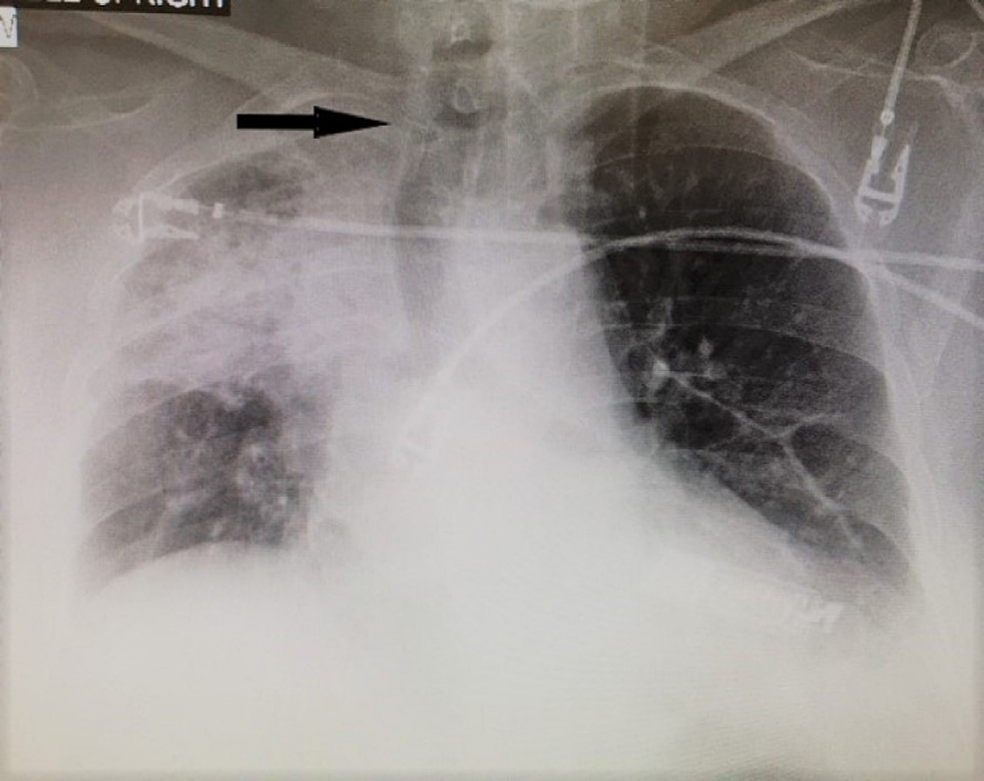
Chest X-ray showing near-total opacification of the right upper lobe with atelectasis and tracheal deviation towards the right (arrow)
